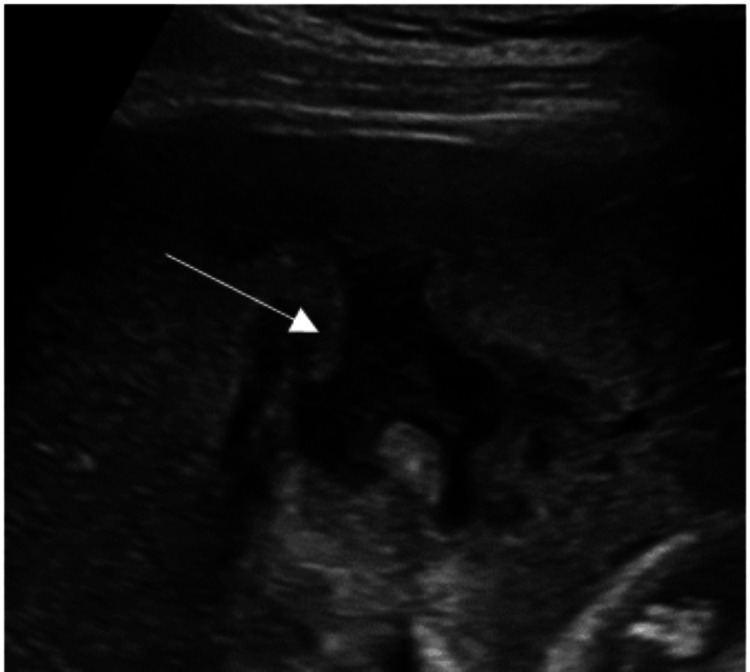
Follow-up ultrasound showing an increase in the size of the abscess.
